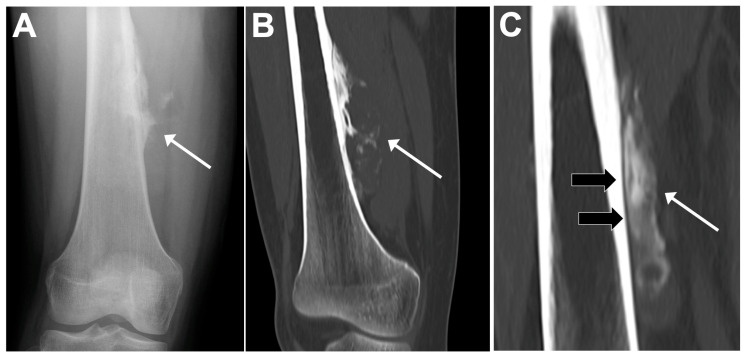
Parosteal osteosarcoma of the distal diaphysis of the femoral bone of a 20-year-old woman. (A) Conventional radiograph (AP projection) showing a bone-forming tumor at the surface of the diaphysis (white arrows). A CT scan can be useful to better depict the relationship to the cortex and periosteum (B,C). In particular, a thin radiolucent cleavage line separates the tumor from the bone cortex (black arrows).
